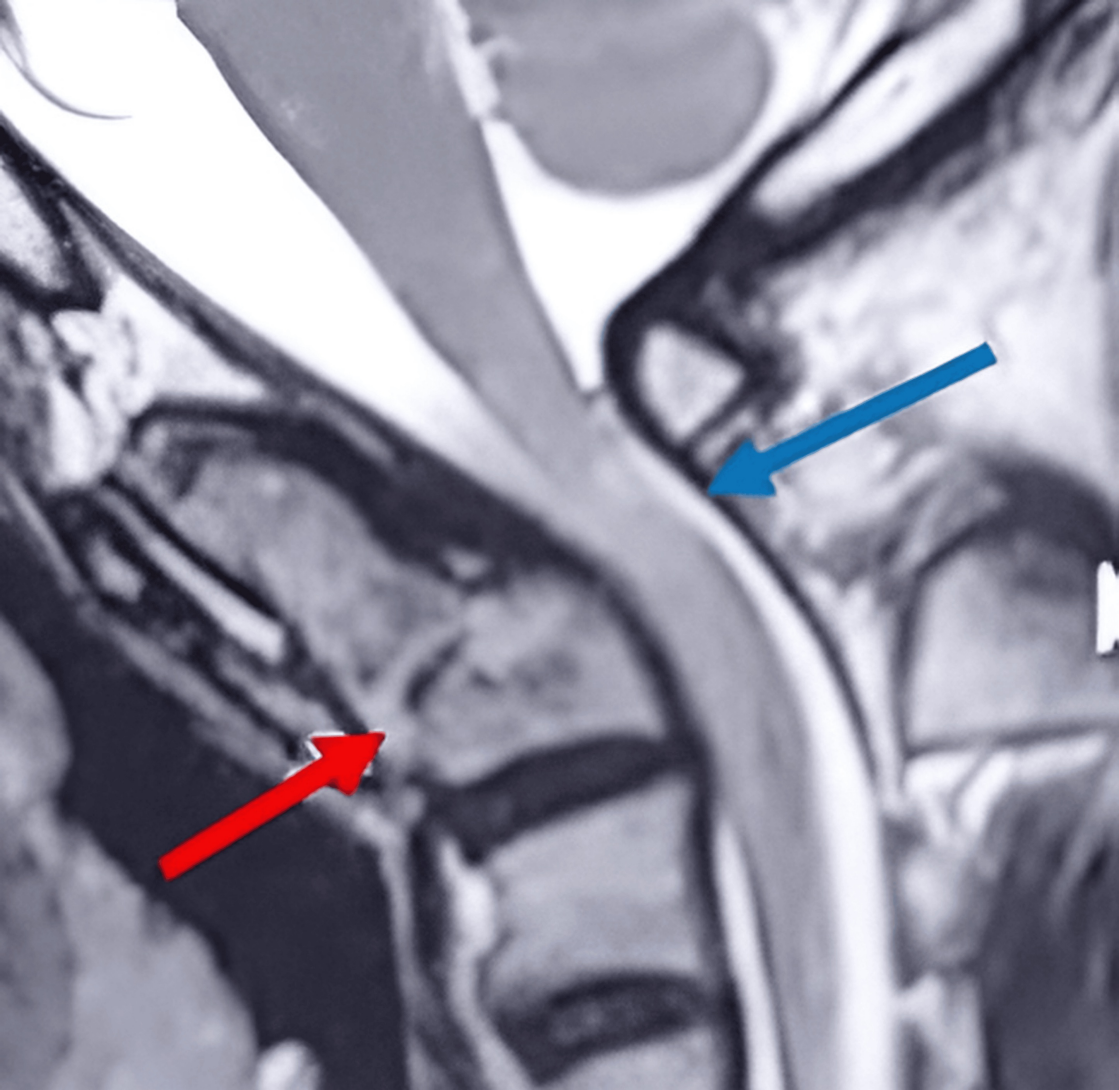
The MRI of the third case shows a type II odontoid fracture, consolidated in a vicious position (red arrow) with cervical kyphosis and complicated by myelopathy (blue arrow).
